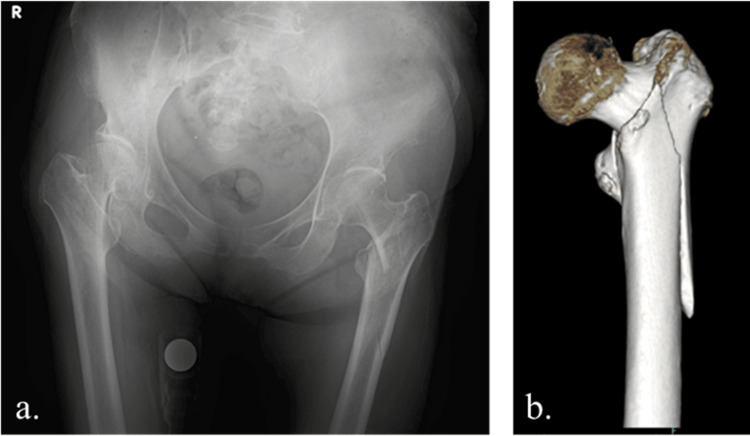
Preoperative X-ray and three-dimensional computed tomography image. a. Preoperative X-ray image. b. Preoperative three-dimensional computed tomography image. A proximal femur fracture was observed in the left hip.
